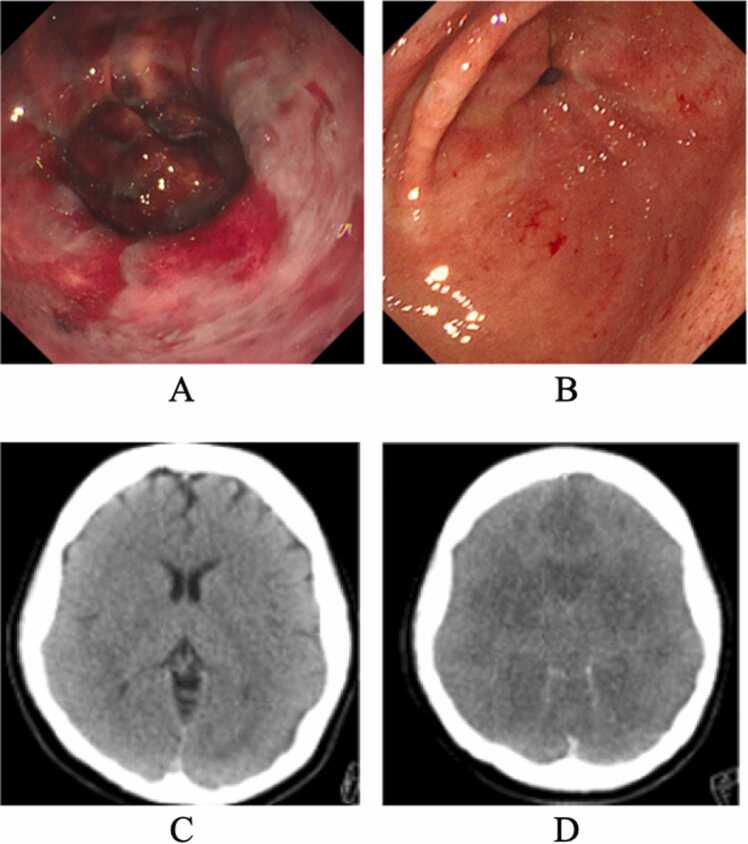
Clinical imaging findings. (A) Upper gastrointestinal endoscopy performed around 04:30 on day 0 (approximately 2 h after ED arrival and ∼70 min after sustained ROSC), revealing extensive hemorrhagic erosions in the gastric antrum. (B) Follow-up endoscopy on day 3 showing marked mucosal healing. (C) Non-contrast head CT obtained at 03:30 on day 0 (10 min after sustained ROSC) demonstrating diffuse cerebral edema. (D) Follow-up head CT on day 8 showing progression to brain herniation.
